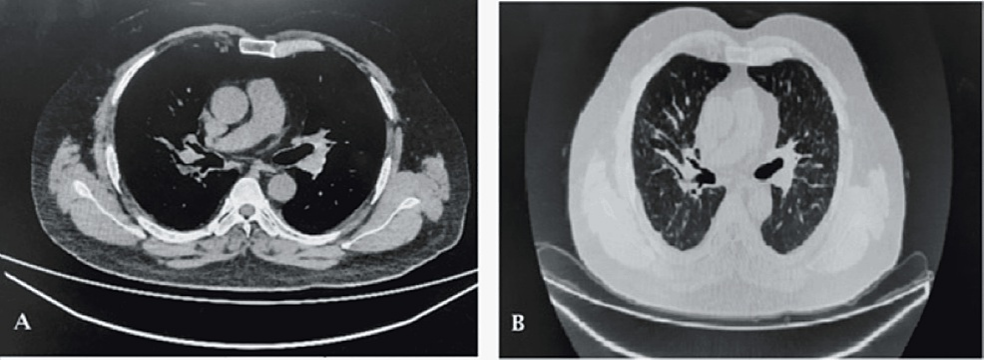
(A) CT thorax showing resolution of the right rib cage collection. (B) CT thorax (lung parenchymal window) showing resolution of consolidation and nodularity.
